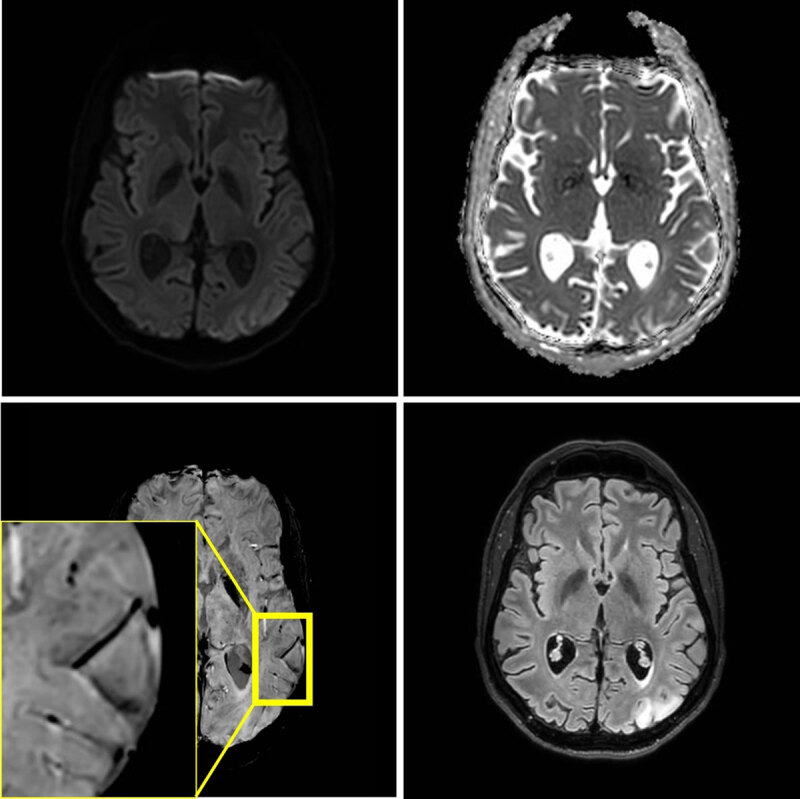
MRI at 3T performed three weeks later, because of a second stroke-like event. A similar lesion in the gyri of the left occipital lobe is present. Signal abnormalities in the previously affected area nearly normalized, but microhemorrhages persisted.
